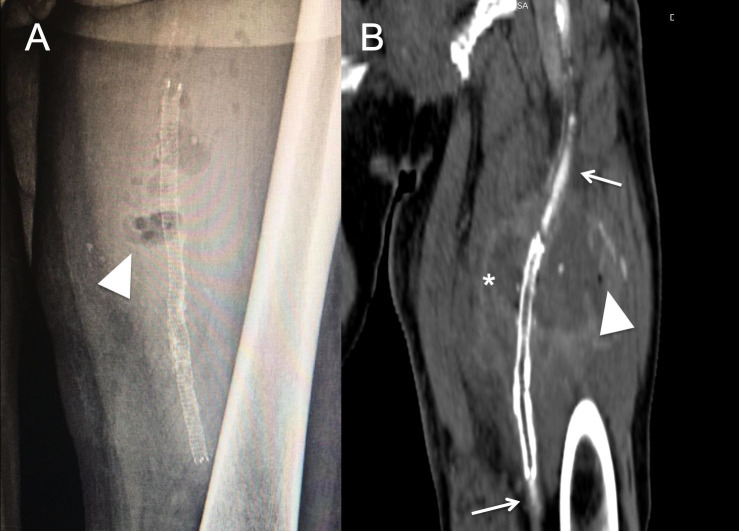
(A) Plain X-ray, stent (arrow) surrounded by air bubbles (arrowhead); (B) Angio CT reconstruction, thrombosed stent (arrow), and large pseudoaneurysm (asterisk).
